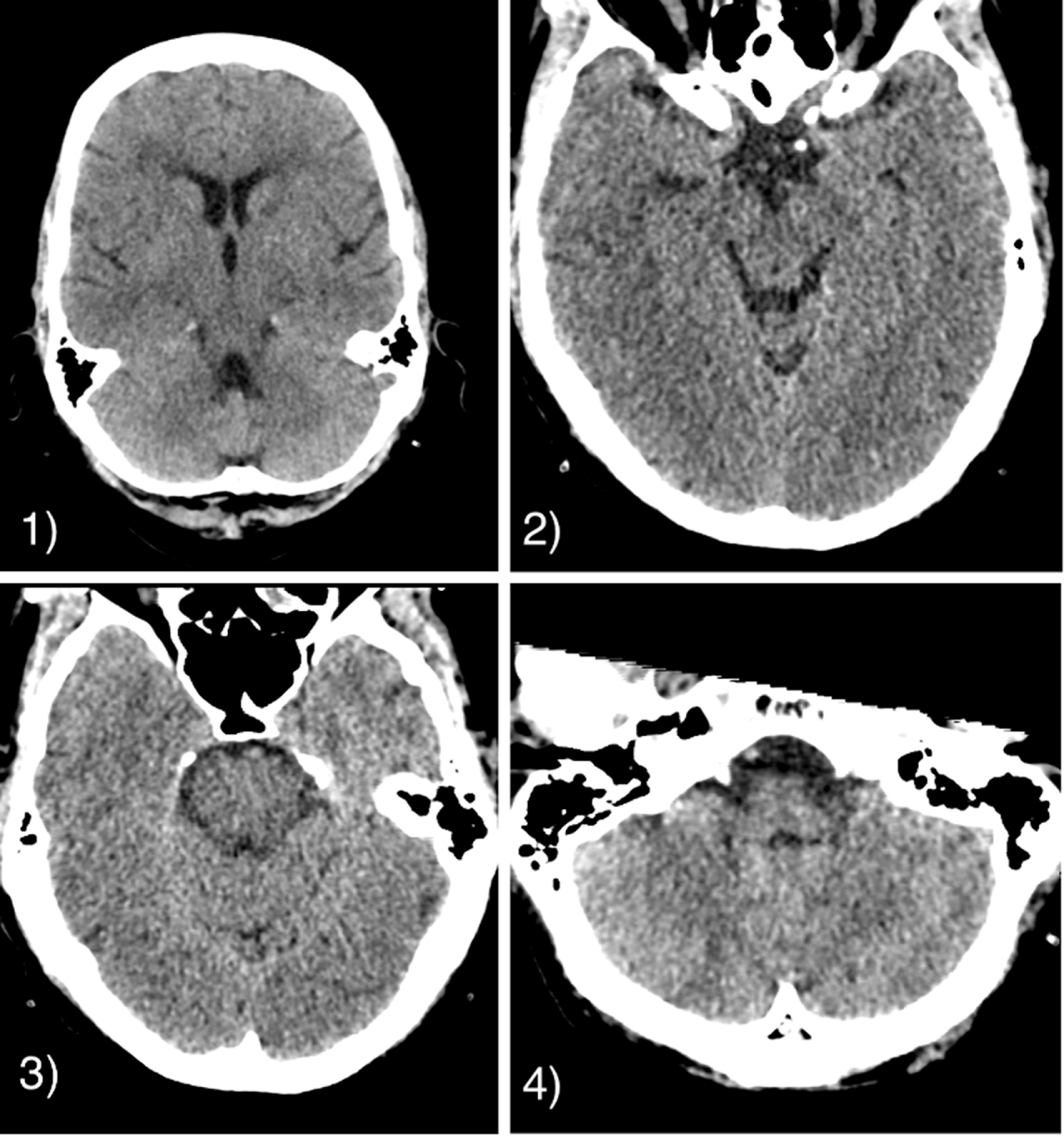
Unremarkable axial CT images one day prior to angiographic treatment of the vertebral artery stenosis. Levels: (1) brain stem (5 mm slice thickness), (2) mesencpehalon, (3) pons, (4) medulla oblongata (all 1 mm slice thickness)
